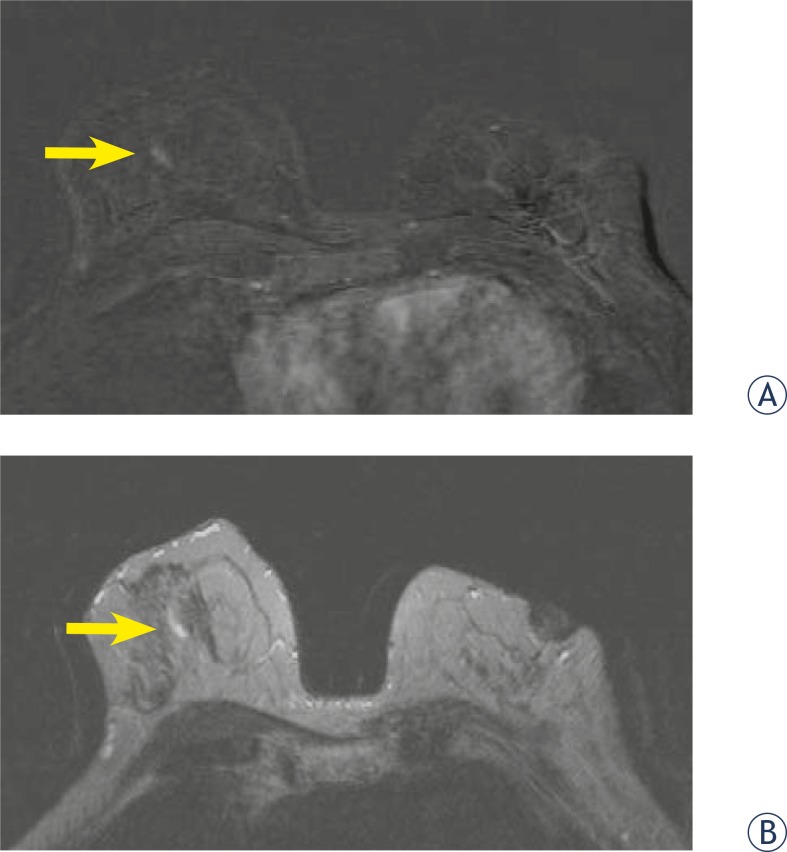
47 year old woman with history of invasive breast carcinoma 10 years ago in her left breast. Retraction of mammila on the right, mammograpic dense breast and benign calcifications. First look and second look ultrasound images showed a cyst. Axial T1-weighted subtracted image after Gadolinium injection (2nd minute).(A) 8×3 mm ductal - linear homogenous, asymmetric enhancement, fast initial increase with postinitial plateau (B) high signal intensity on T2-weighted images, categoriaztion BI-RADS 4. MRI-guided vacuum-assisted biopsy revealed high-grade DCIS withouth microcalcification.
